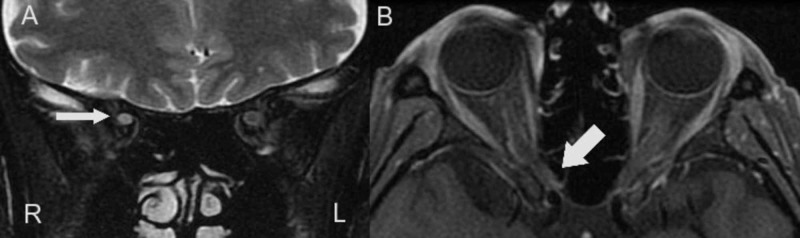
Optic nerve MRI findings. T2-weighted coronal imaging showed that the right optic nerve had atrophied (A). T1-weighted axial imaging showed that the right optic nerve was actively enhanced near the apex of the orbit region (B).
